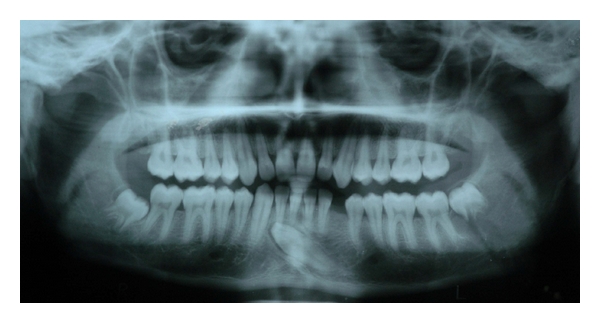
Panoramic radiograph showing transmigrated 33 lying below the apices of mandibular incisors.
